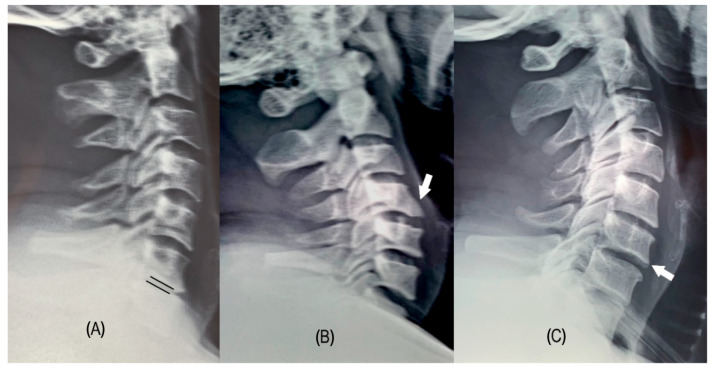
Examples of cervical degeneration: (A) A lateral cervical radiograph showing decreased disc space between parallel black lines, compared with adjacent levels. (B) A lateral cervical radiograph with an arrow indicating an anterior osteophyte at the C4 vertebral body. (C) A lateral cervical radiograph with an arrow highlighting sclerosis of the inferior endplate of C6. Source: Personal archive.
